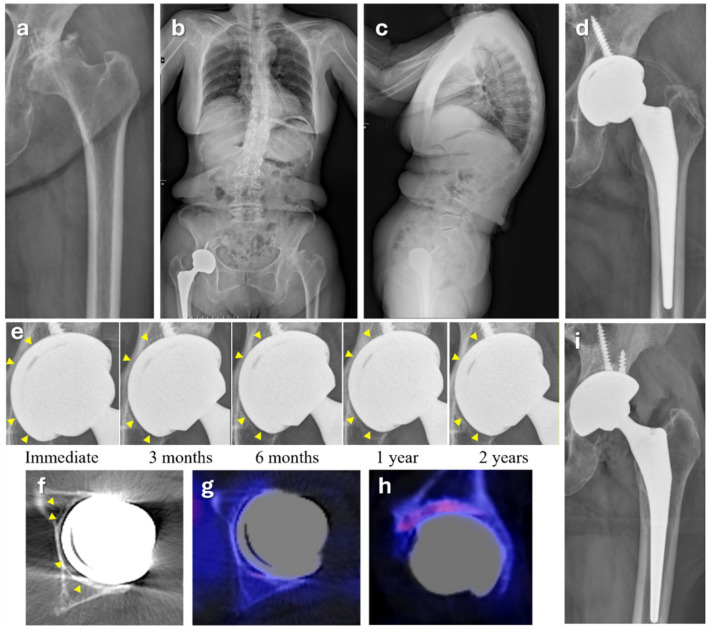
Radiographic and nuclear imaging evaluation of a patient with suspected failure of osseointegration after THA. (a) Preoperative anteroposterior radiograph showing severe arthritic changes in the hip joint with femoral head destruction. (b,c) Standing anteroposterior and lateral whole-spine radiographs demonstrating degenerative spinal imbalance. (d) Immediate postoperative radiograph of the left hip. (e) Serial magnified radiographs of the acetabular component from immediately after surgery to 2 years postoperatively. Yellow arrowheads indicate persistent radiolucent lines around the cup–bone interface, suggesting a lack of osseointegration. (f–h) SPECT/CT images revealing a radiolucent gap around the acetabular component, with increased uptake at the superior aspect of the acetabular component, indicating mechanical loosening. (i) Postoperative radiograph after revision.
